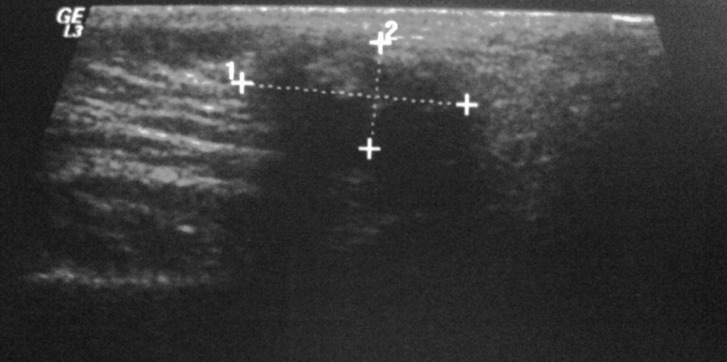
Ultrasound examination showing heterogeneous echotexture of the left parotid but without acute inflammatory foci.
